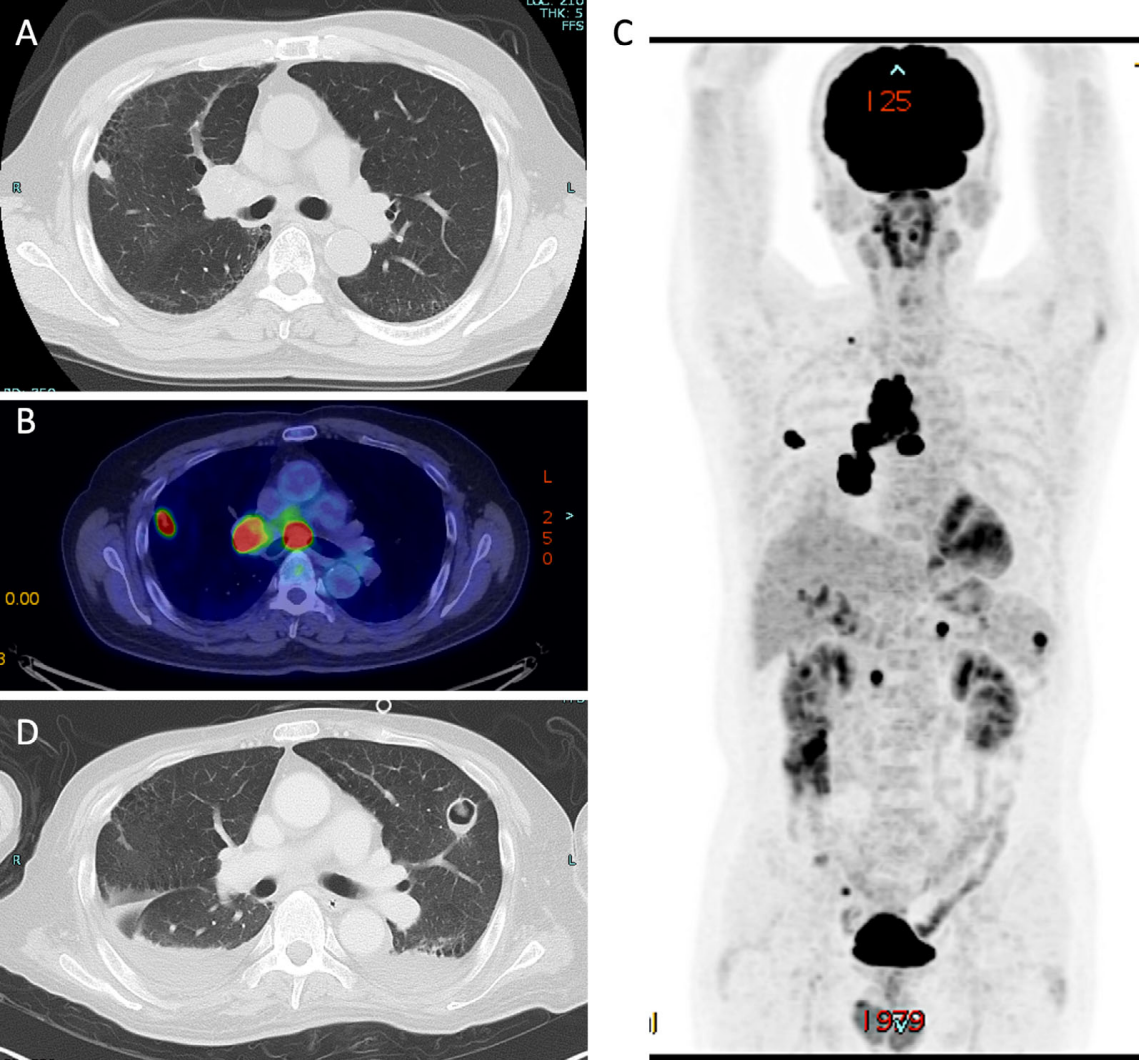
Radiological findings of the lung cancer. (A, D) Chest CT reveals pulmonary opacity in the upper lobe of the right lung three months before admission. (B, C) PET‐CT reveals hot spots in the right lung, lymph nodes of the mediastinum, spleen, and ilium. (D) Chest CT at the patient’s admission reveals a reduction in size of the lung tumor.
